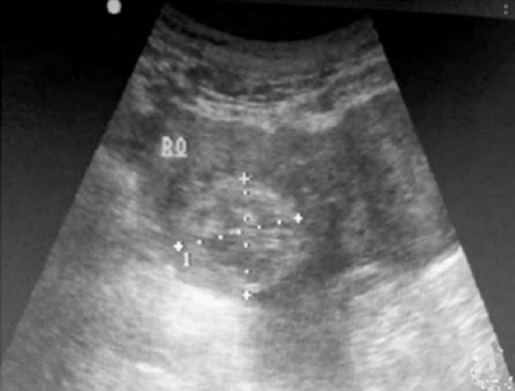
Results of transabdominal sonography: ectopic gestation mass with enhanced echo in adnexa area.
